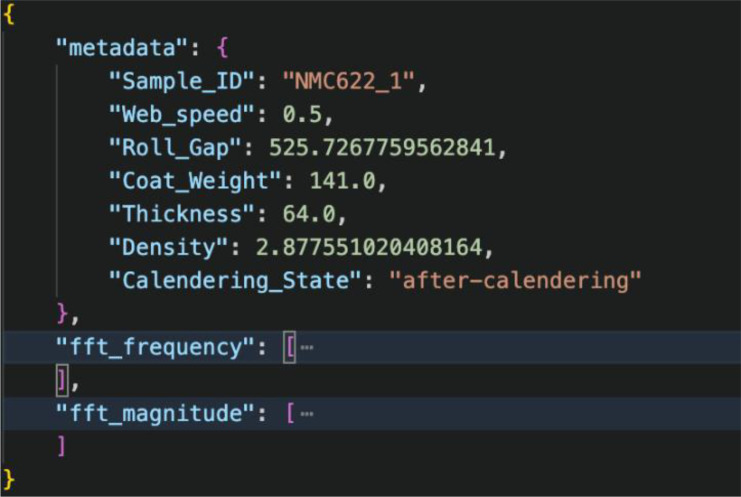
Sample JSON structure for a cathode electrode (NMC622_1), showing the stored metadata together with the FFT frequency array and corresponding magnitude spectrum derived from the ultrasonic measurement.
